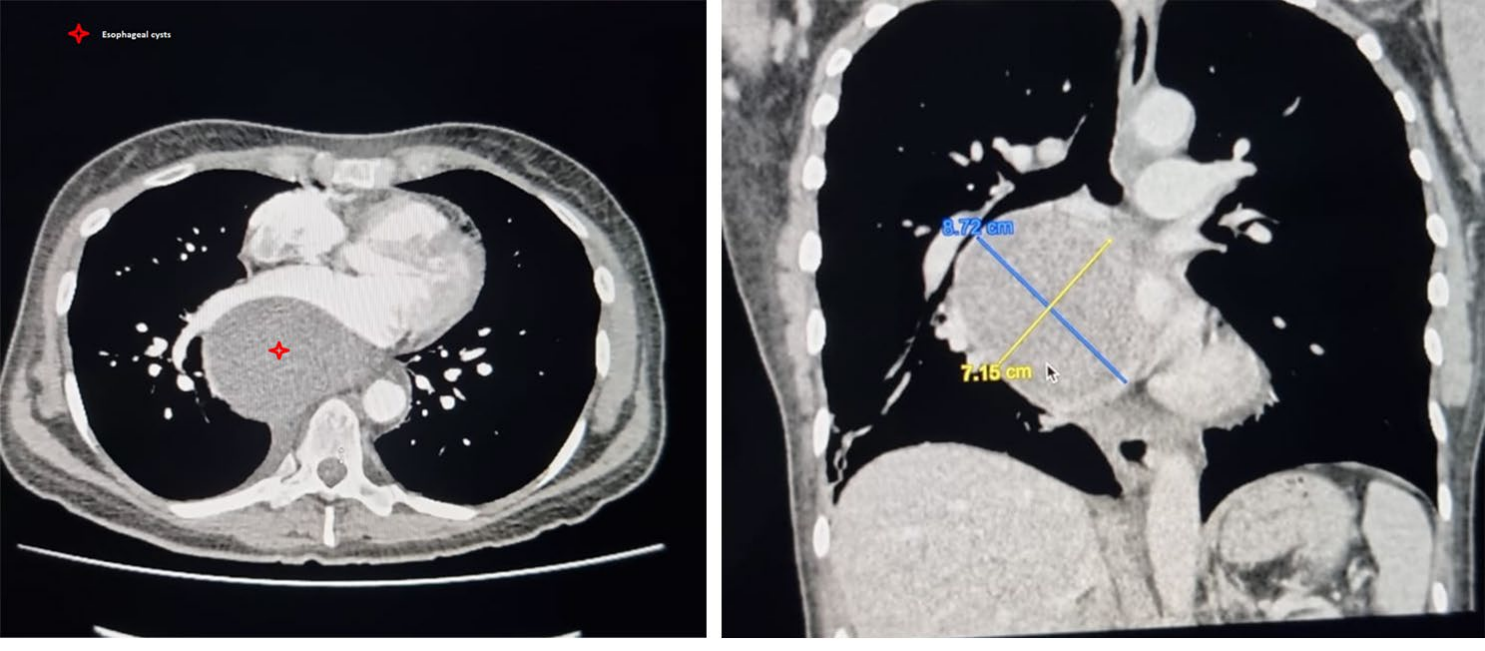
A: CT, The mass in the mediastinum besides the esophagus. B: CT, Cystic Mass displacing the organs
